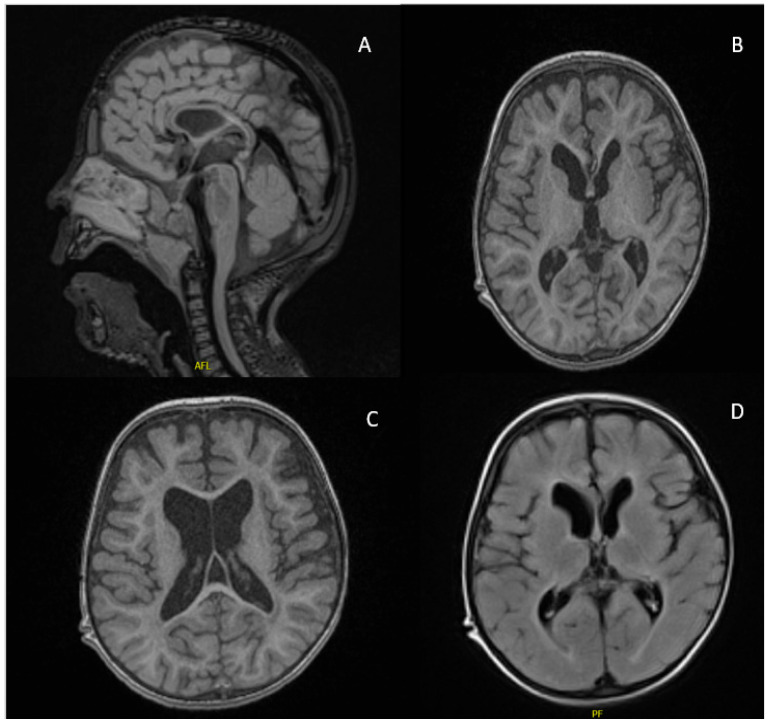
Brain MRI findings of the patient at the age of 3 years showing corpus callosum thinning ((A), T1), hypomyelination, and brain volume loss ((B,C) (T1), and (D) (T2)).
